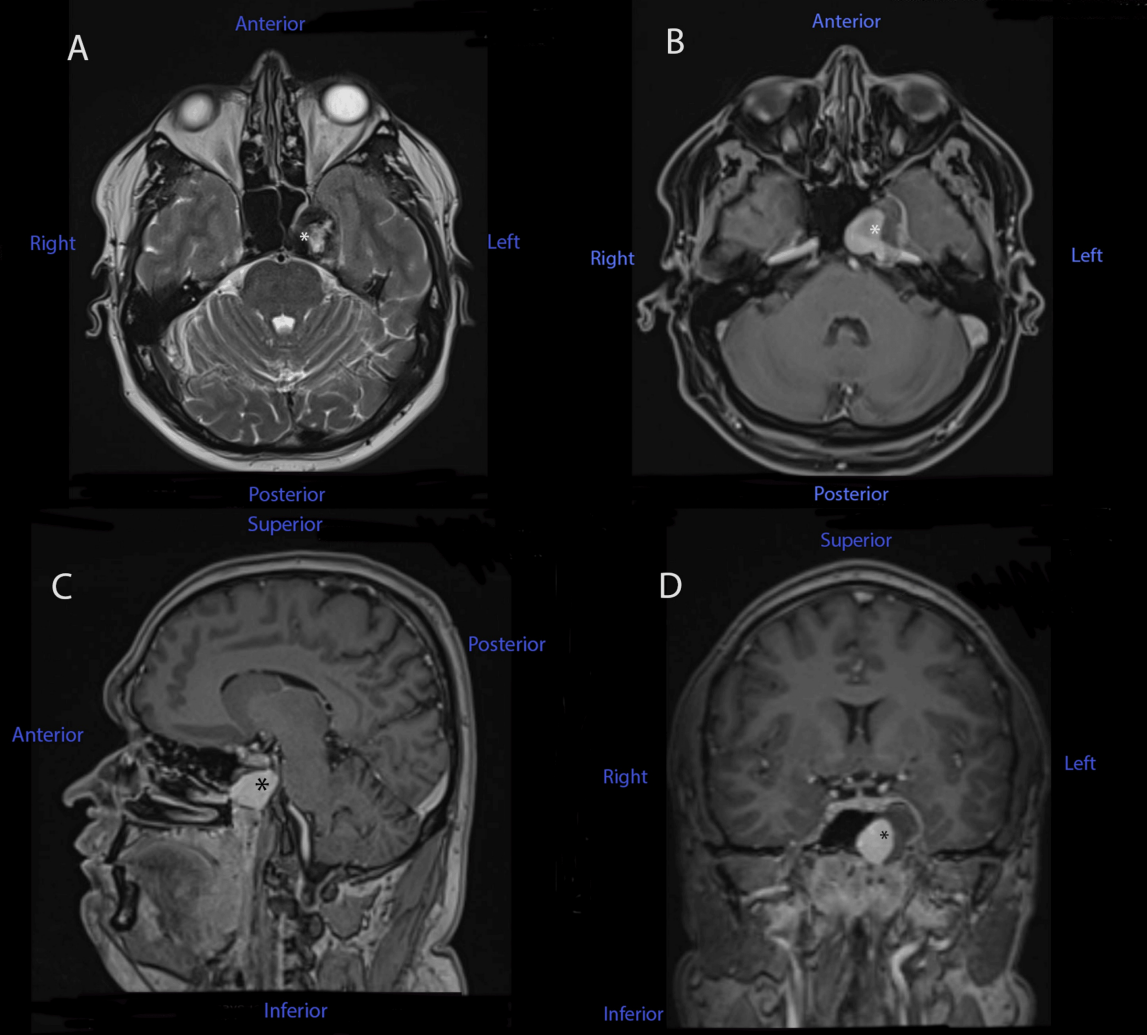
MRI brain images of our patient, before being admitted to our hospital. (A) T2-weighted axial sequence; (B) T1-weighted contrast-enhanced axial image; (C) T1-weighted contrast-enhanced sagittal image; (D) T1-weighted contrast-enhanced coronal sequence. Note that the asterisk marks the presence of the lesion in each sequence.
